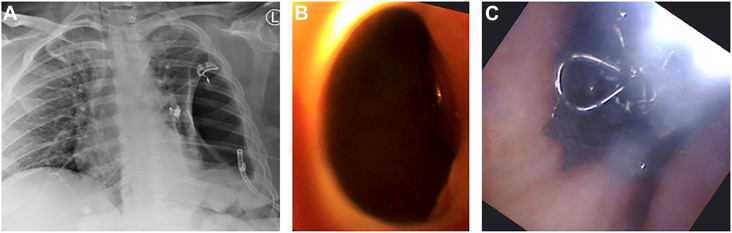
The left upper lobe was partially collapsed on the preoperative chest x-ray (A). The Ion (Intutive) bronchoscope was directed to the opening along the bronchial staple line (B) where a combination of sealant and coils were deployed (C) for closure.
